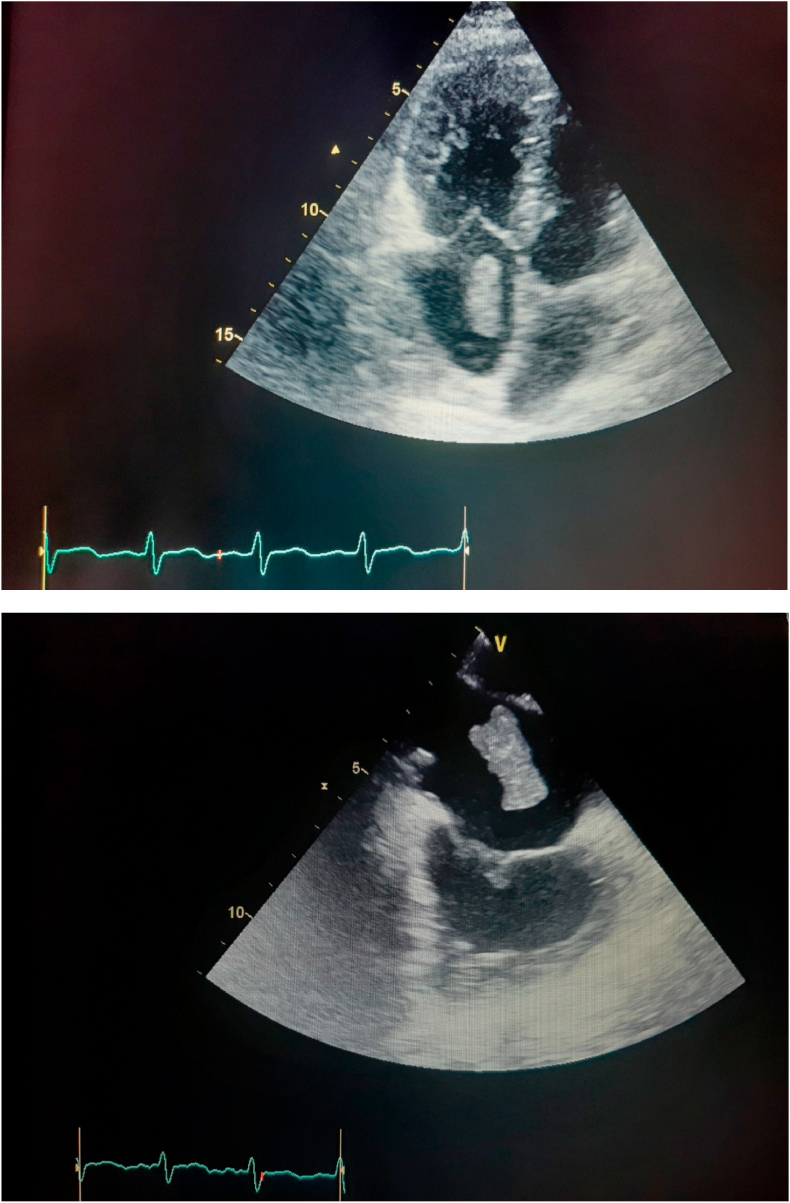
Thransthoracic (A) and transesophageal (B) echocardiography images showing a highly mobile right atrial thrombus.
